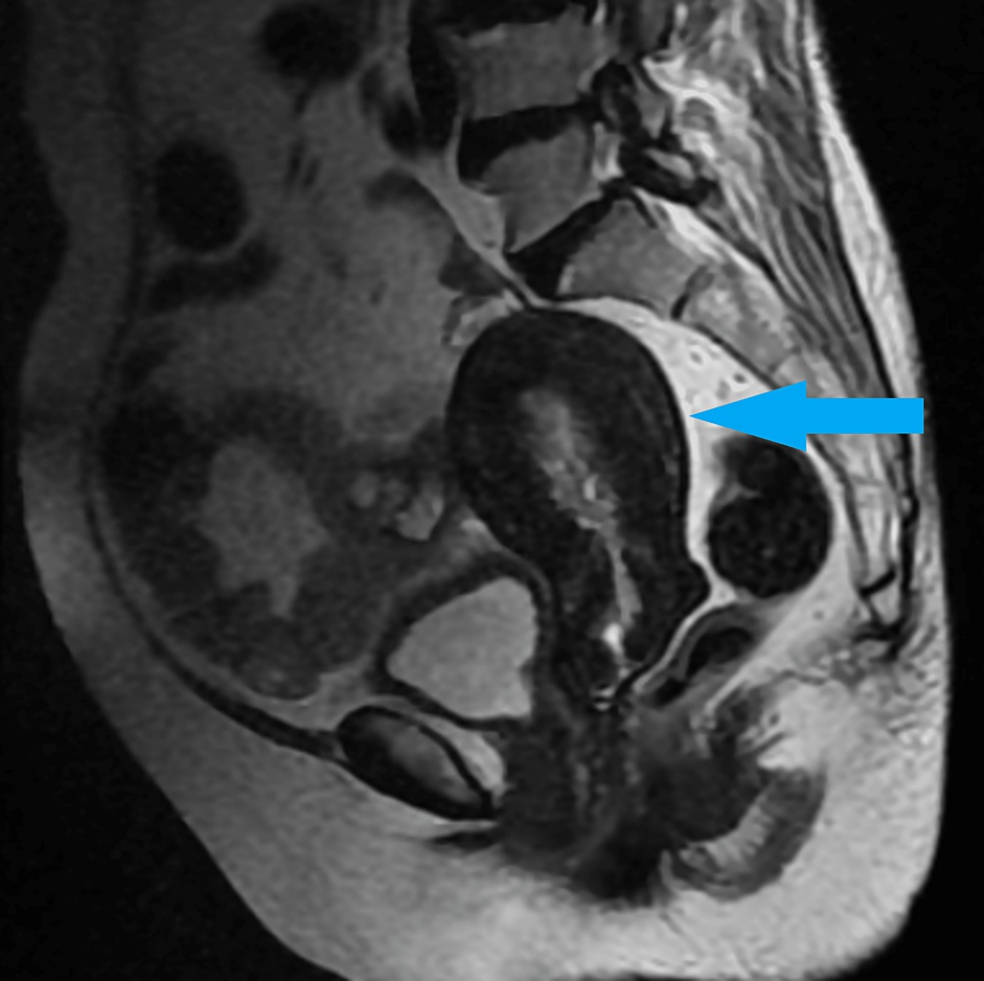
Magnetic resonance image showing a sagittal section of uterus (blue arrow)
